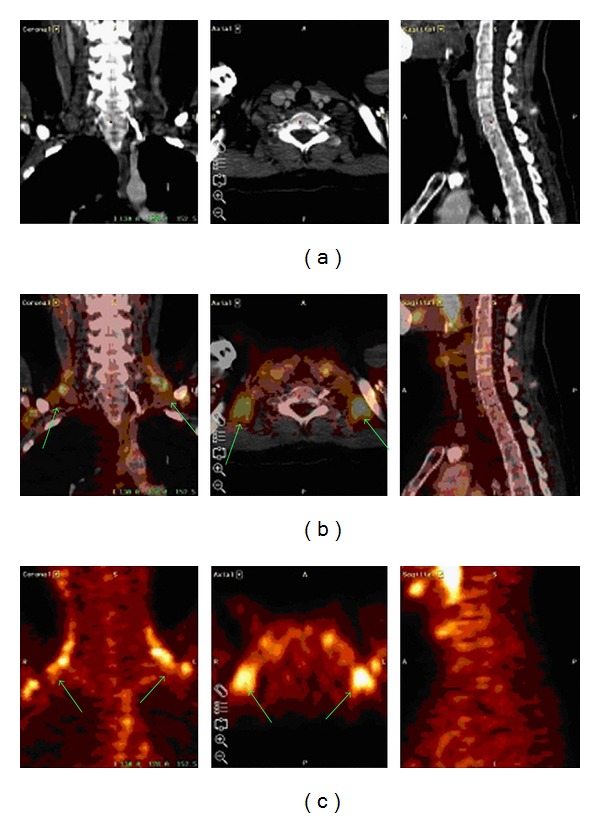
Axial, coronal, and sagittal reformatted images of a patient showing a typical “pitfall” with F18-FDG PET (third row). A positive bilateral cervical activity could be identified as activated brown fat tissue by using image fusion (second row). first row: computed tomography image; second row: software-based image fusion; third row: F18-FDG PET.
